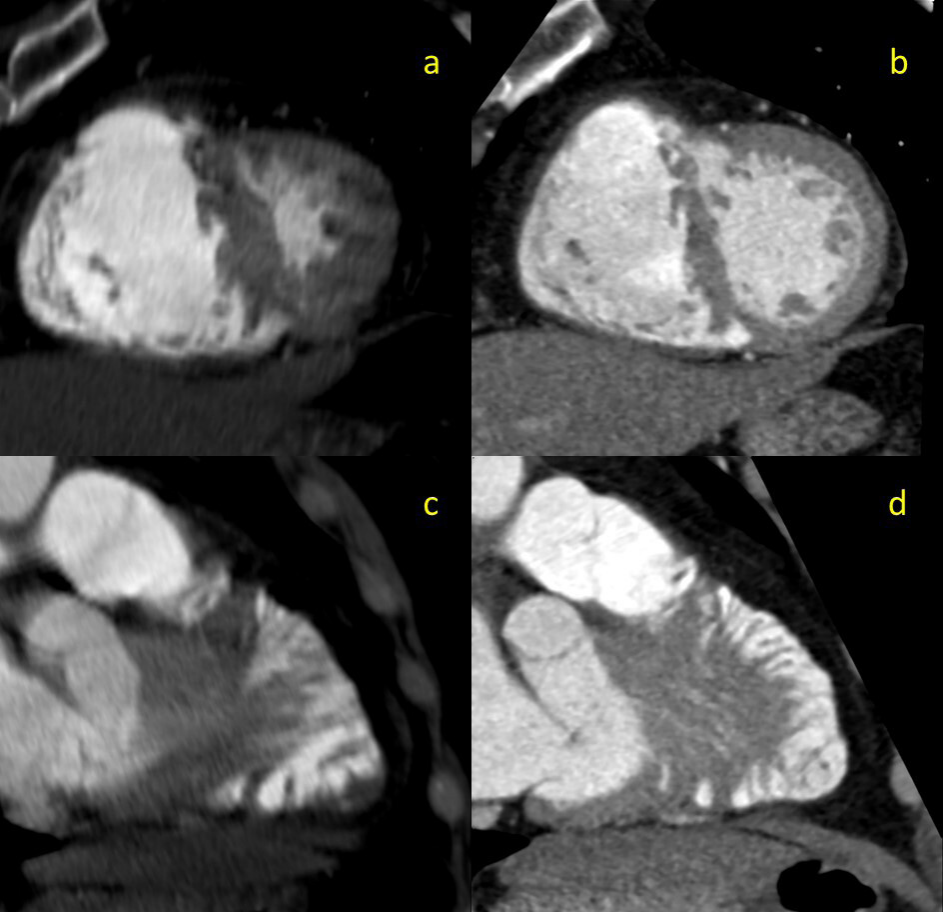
2-mm thick multiplanar reformation (MPR) images of the interventricular septum in cross-sectional (a, b) and lengthwise (c,d) orientations. Images on the left (a,c) are derived from the initial non-gated CTA. Images on the right (b,d) are derived from the follow-up gated-CTA exam
